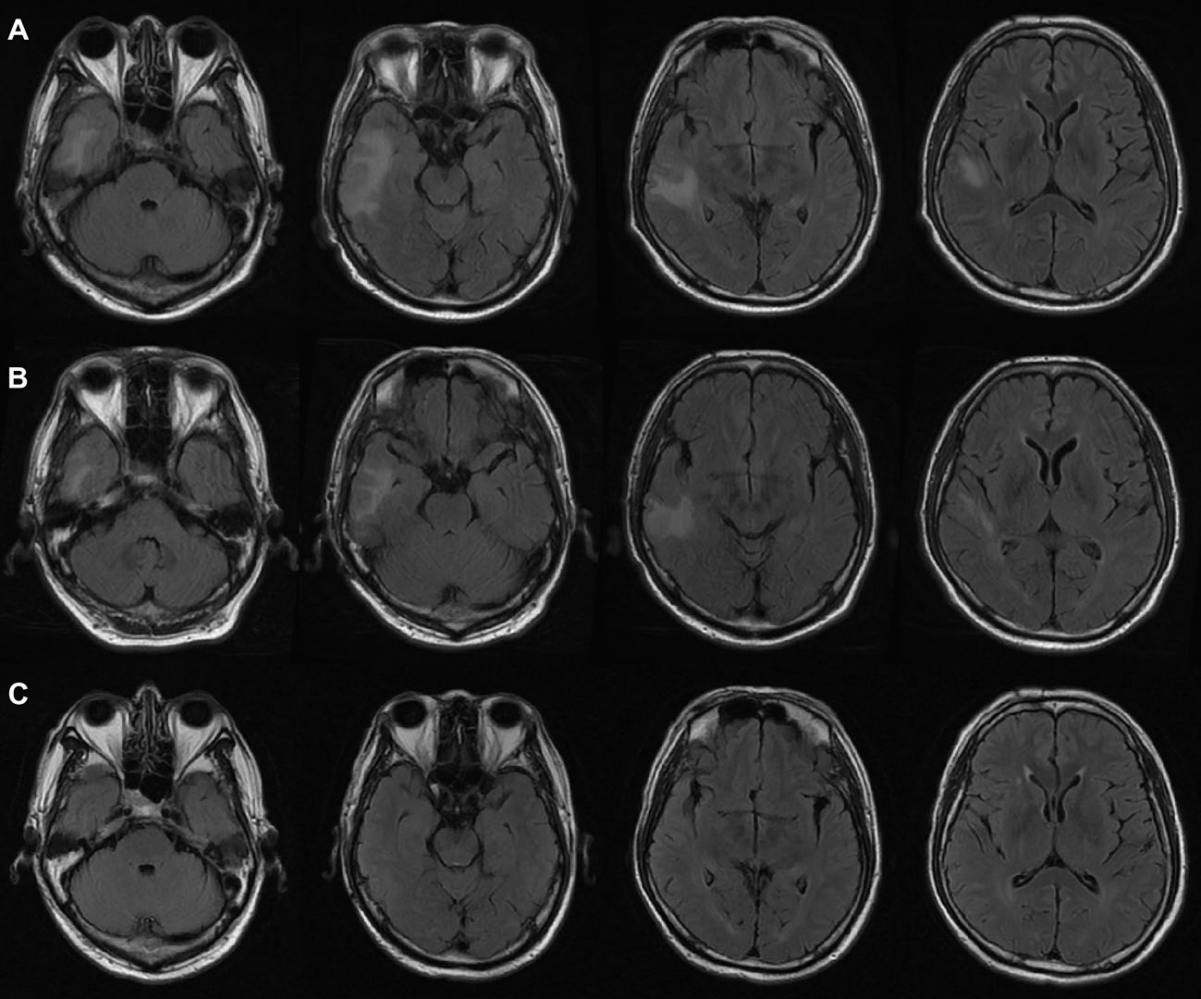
The imaging changes before and after immunosuppressive therapy. A Brain MRI showed the first finding of a T2-FLAIR hyperintense signal in the right temporal lobe. B Brain MRI showed the focal lesion seemed to diminish after the first 12 days of methylprednisolone, although neurologic symptoms appeared. C The latest brain MRI showed that the T2-FLAIR hyperintense signal was resolved
